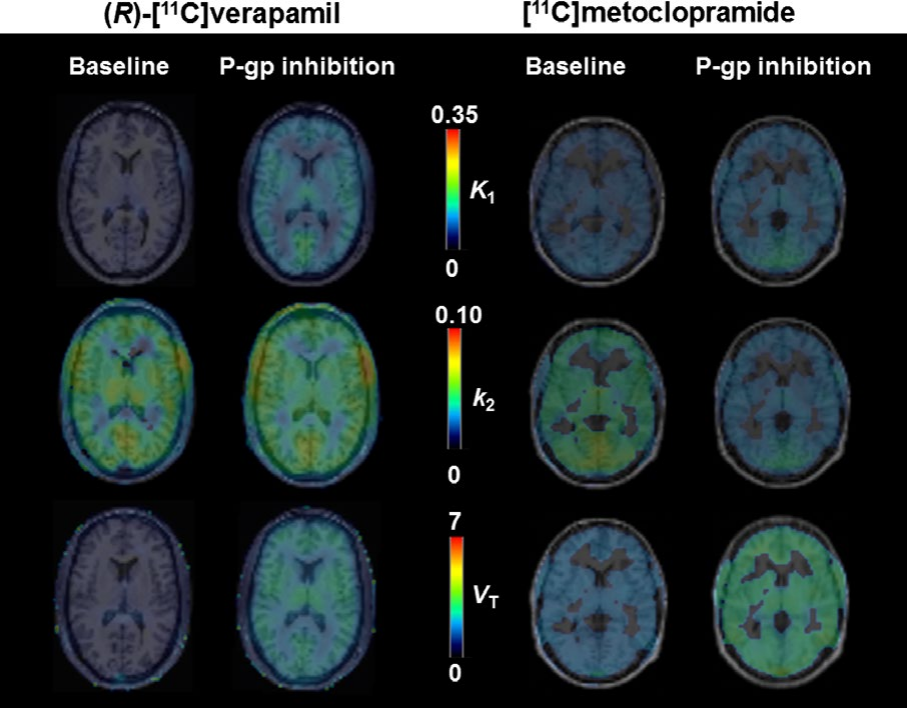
Representative magnetic resonance imaging‐co‐registered parametric positron emission tomography (PET) images in one subject each for (*R*)‐[^11^C]verapamil (left) and [^11^C]metoclopramide (right) at baseline and after P‐glycoprotein (P‐gp) inhibition either with tariquidar (infused during the PET scan at 2.3 mg/kg/hour) or with cyclosporine A (infused during the PET scan at 2.5 mg/kg/hour). In the parametric images, the intensity scales represent the outcome parameters of kinetic modeling (*K*
